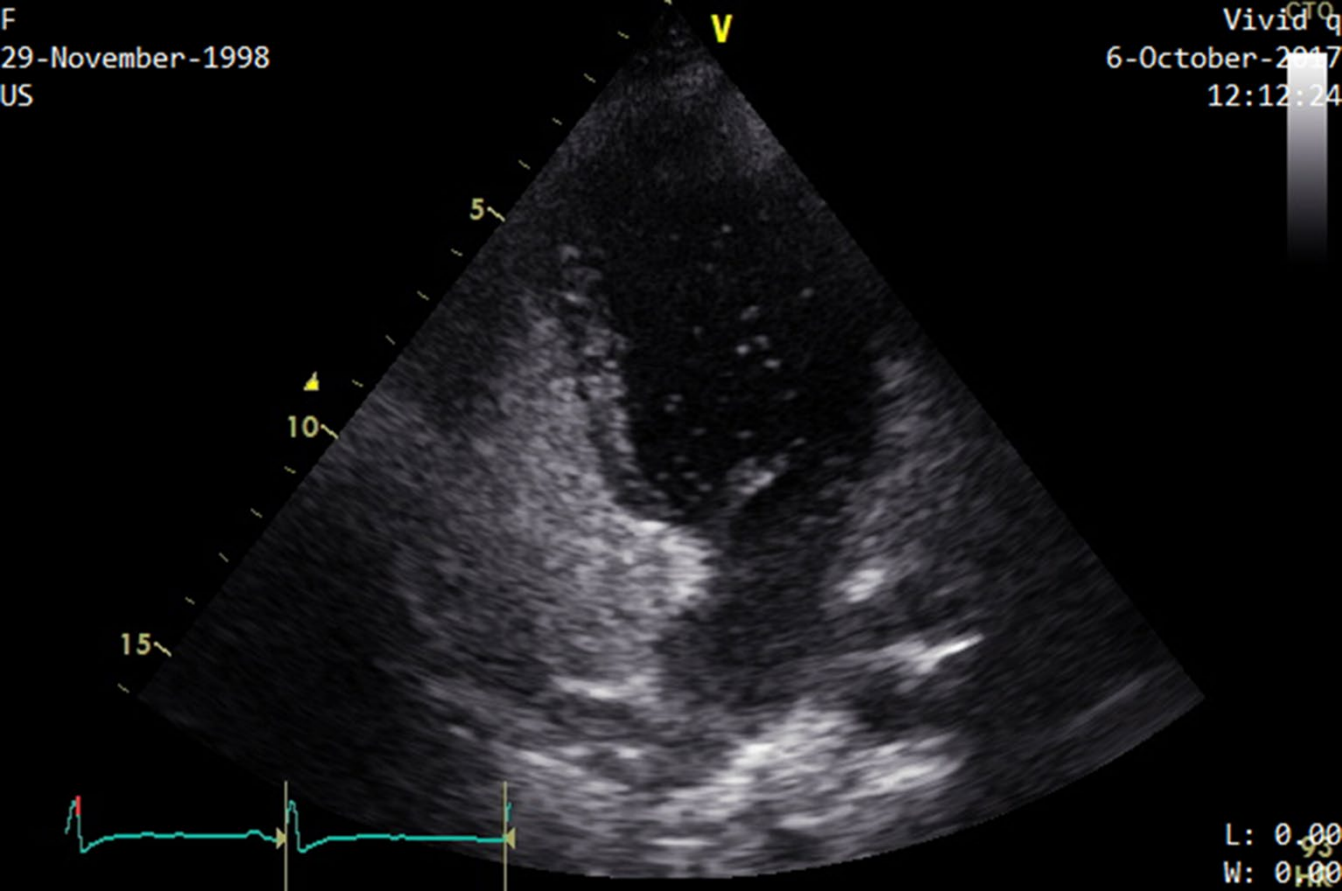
Transthoracic echocardiographic evaluation while the patient was normally breathing shows early microbubbles in left cardiac cameras
